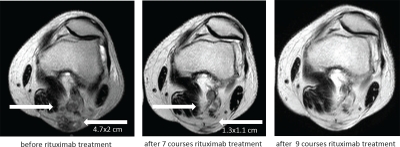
Complete regression of the metastases in the left popliteal region during rituximab therapy Popliteal metastases were screened by MRI before the start of rituximab treatment (day -28) and after 7 and 9 courses of local treatment. The popliteal bulk of metastases measured 4.7 × 2 cm before therapy and regressed to 1.3 × 1.1 cm and disappeared after 9 courses of treatment (right arrow). Left arrow indicates a solitary metastasis which completely disappeared after 7 courses. Complete remission was stable.
